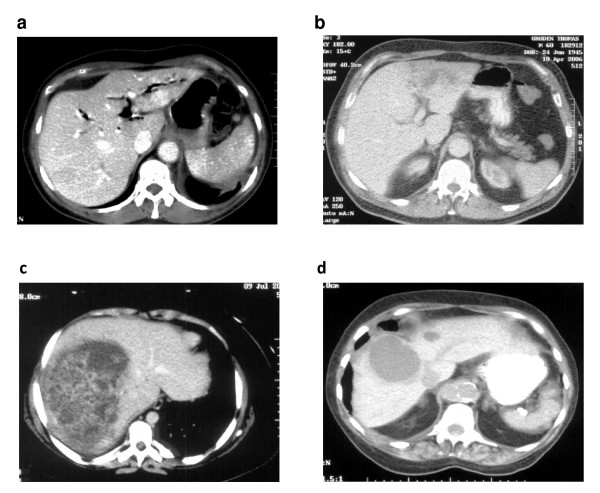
(a-d) CT images of four patients with hepatic abscesses.
